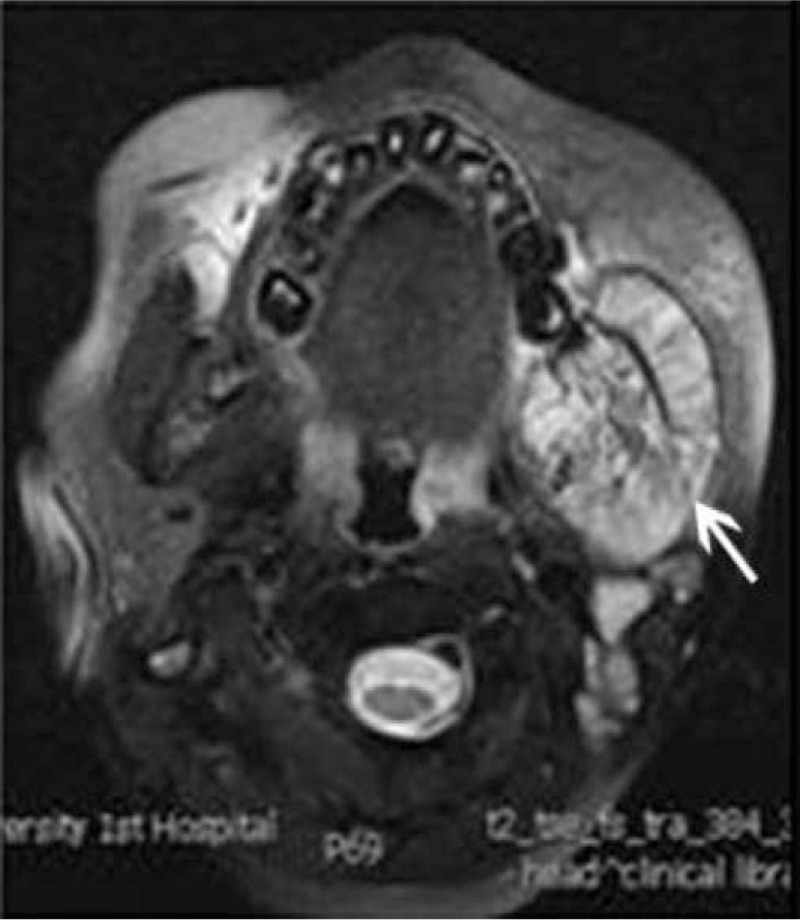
Magnetic resonance imaging scan showed extension of a mass in the left side of the mandible with a clear osseous destruction before radiotherapy.
